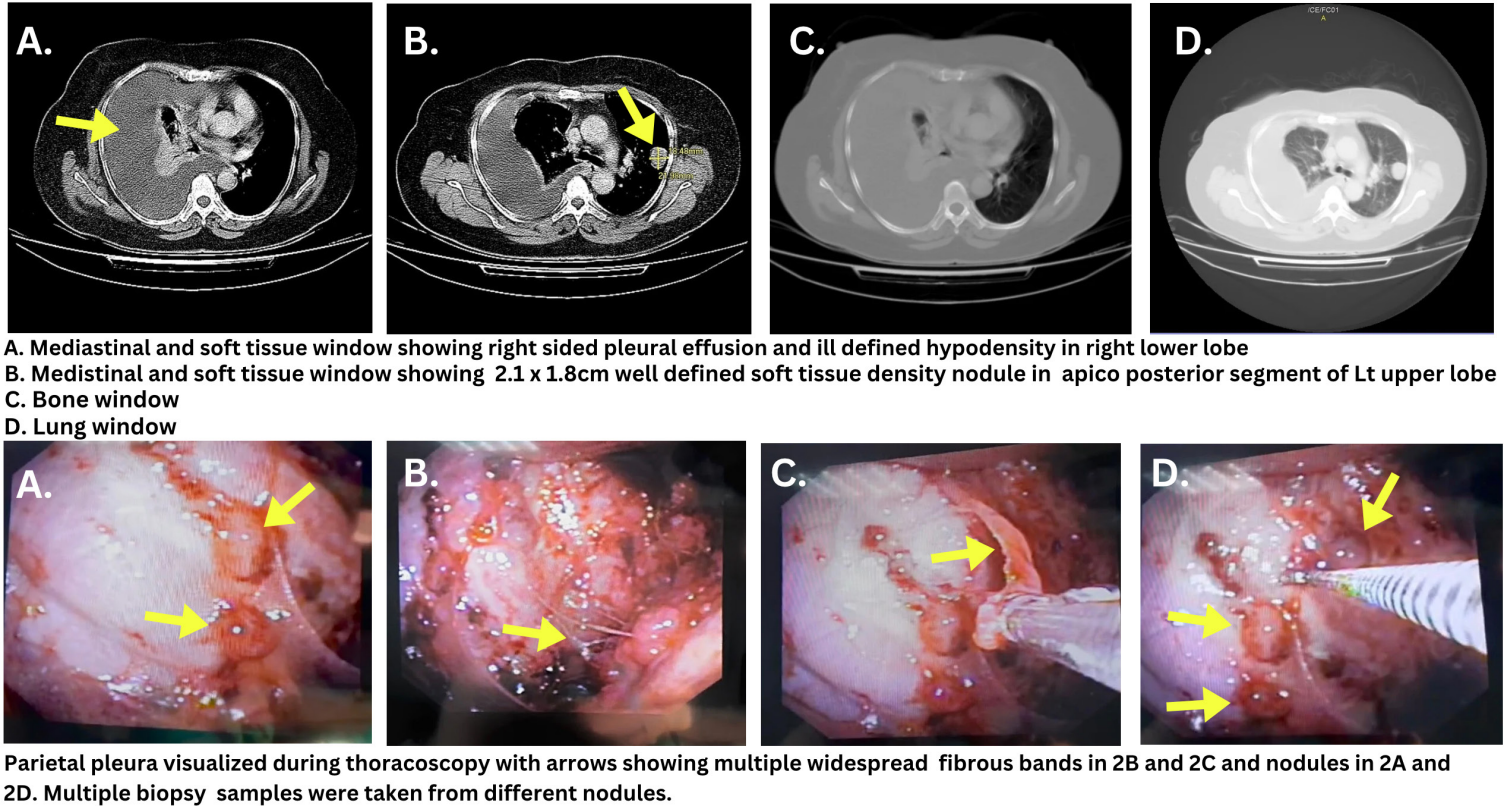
Axial contrast‐enhanced chest CT with corresponding thoracoscopic views showing moderate right‐sided pleural effusion with collapse consolidation in the right lower lobe, along with a solitary soft tissue nodule in the left upper lobe. Thoracoscopy revealed multiple widespread pleural nodules, from which biopsies and brushings were obtained.
